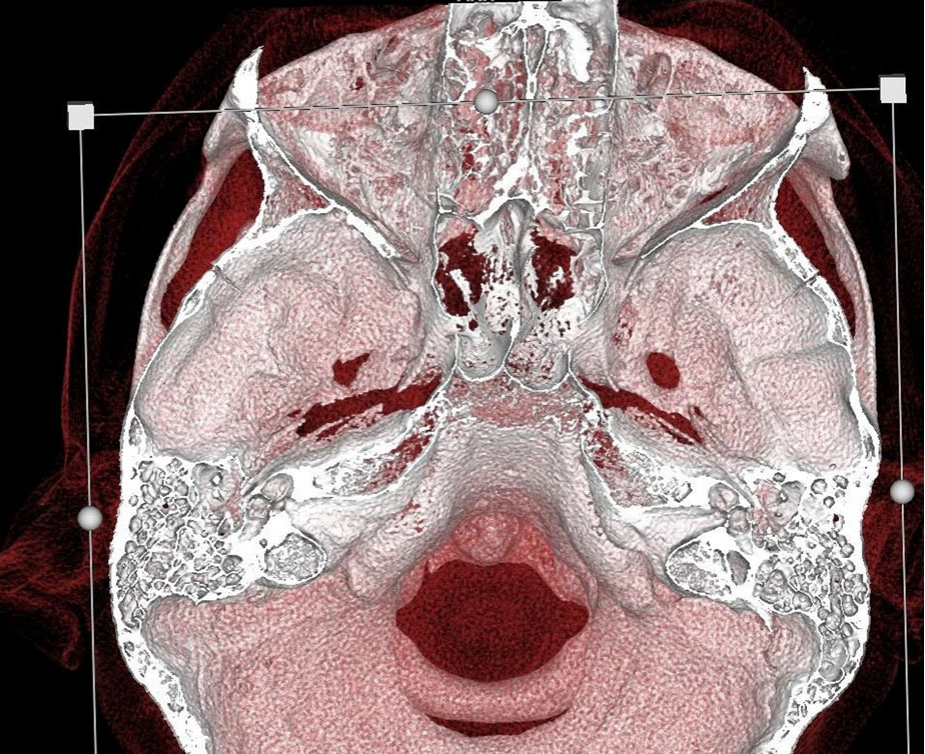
CT- Volume Rendering Techniques scan of temporal bone - Mondini Malformation
